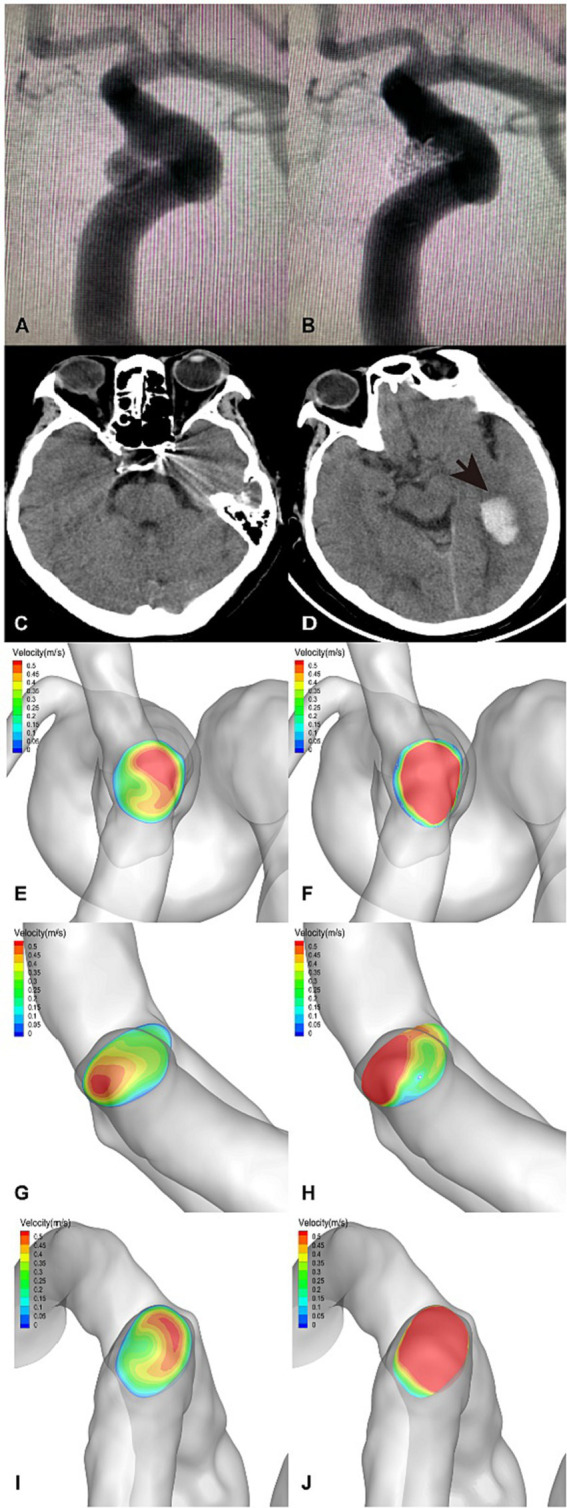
An ophthalmic segment aneurysm of the left ICA in DICH of type 2 was treated with EP2 stent assisted coiling. (A) Preprocedural angiography of the aneurysm and (B) postprocedural immediate angiography revealed Raymond grade 1. (C) The coiled aneurysm was showed by CT scan at 24 h later after the treatment. (D) CT scan obtained 3 days post-procedure, revealing ipsilateral temporal lobe DICH (arrow). A significant increase in velocity was observed on the terminal section of the ICA (F) compared to the preoperative results (E) by computer hemodynamic detection (Increase rate was 47.4%). The velocity of DICH related artery (MCA) increased significantly after embolization with an increase rate of 77.0% (I,J), while the flow velocity of DICH unrelated artery (ACA) increased with increase rate of 20.7% (G,H). The imbalance index of this patient was 56.3%.
